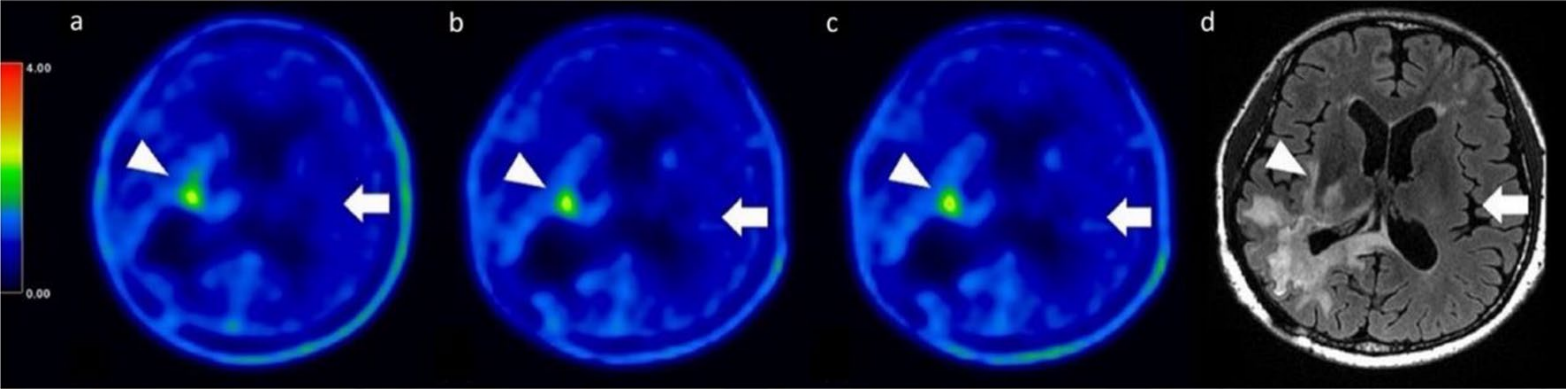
(a–d) Representative images of the 3 AC methods. While visual uptakes in target (tumor) lesion (arrowhead) and normal brain (arrow) showed no differences among PET/CT-AC (a), PET/MR ZTE-AC (b) and PET/MR AB-AC (c) in reference to the T2-Flair MRI (d), the subtle TNR however could be statistically discriminated.
